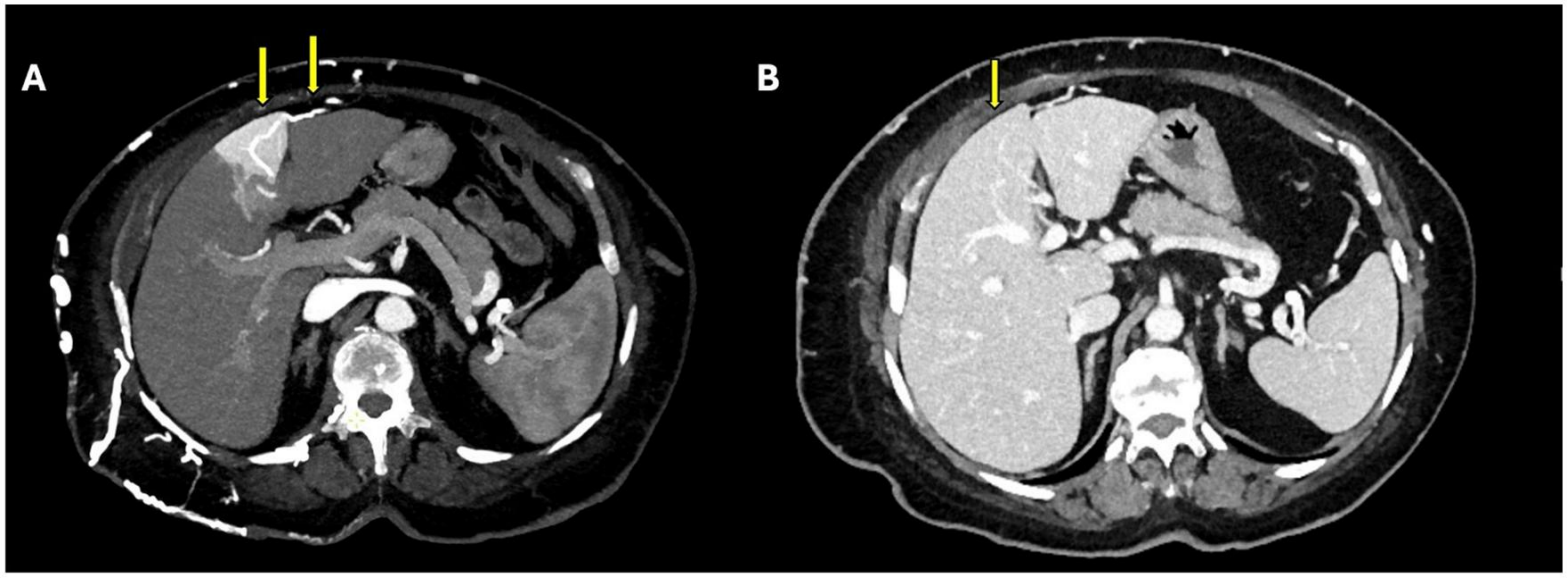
Axial arterial phase (A) and portal phase (B) CT images of a patient under follow-up for renal cell carcinoma. (A) In the arterial phase, the Superior Sappey vein draining into segment 4 parenchyma is observed (arrows). A contrast enhancement area consistent with the “hot spot sign” is visible in segment 4 parenchyma. (B) In the portal phase image, this area appears isodense with the liver parenchyma (arrow). The arterial phase enhancement and isodense appearance in the venous phase are consistent with a pseudolesion.
